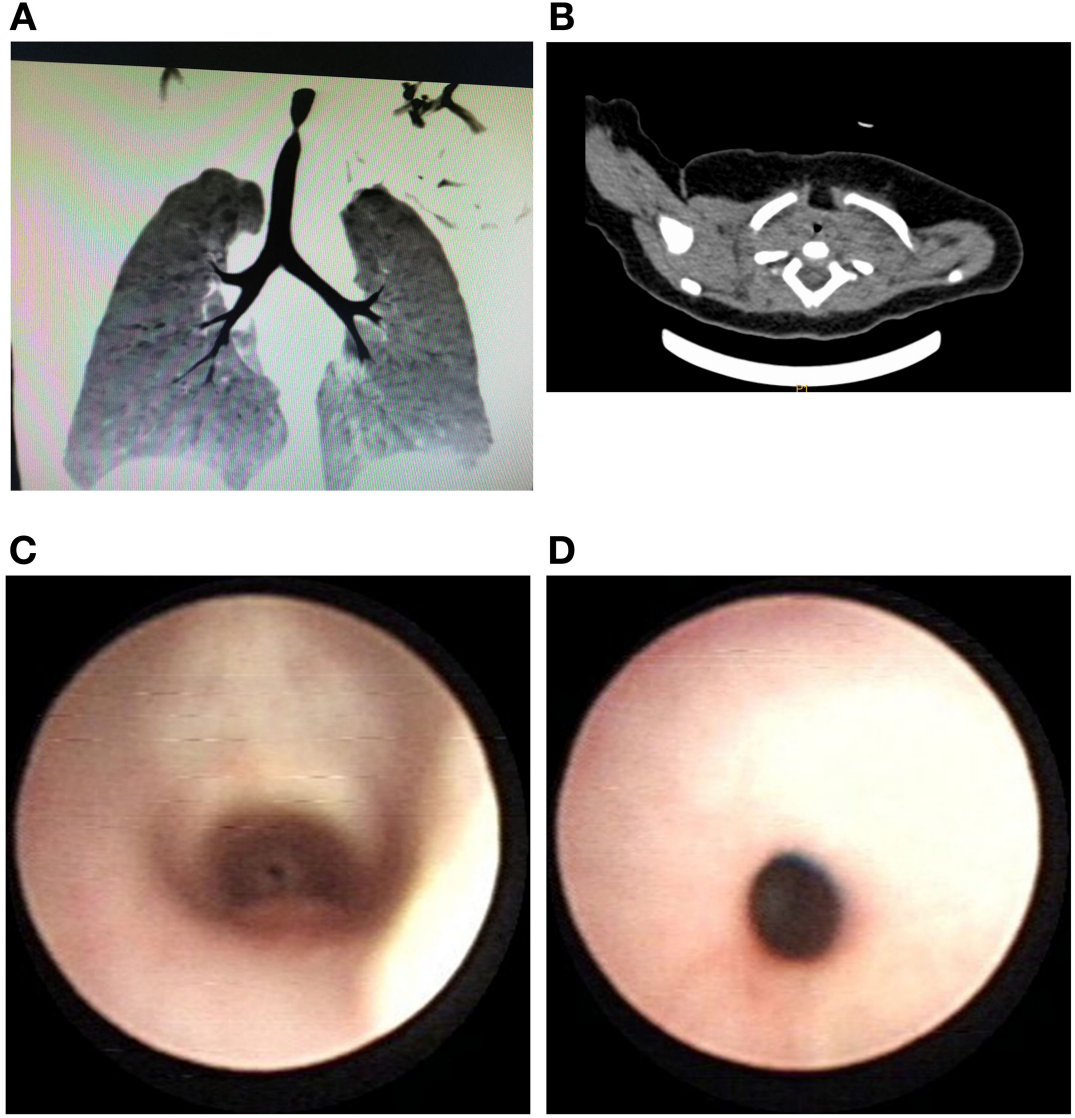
(A) Airway reconstruction shows the localized stenosis of the lumen in the cervical trachea. (B) The irregular narrow lumen of the cervical trachea shown by a CT scan. (C) Cervical stenosis was observed through the subglottic region during flexible bronchoscopy. (D) Bronchoscopy showing segmental concentric stenosis.
